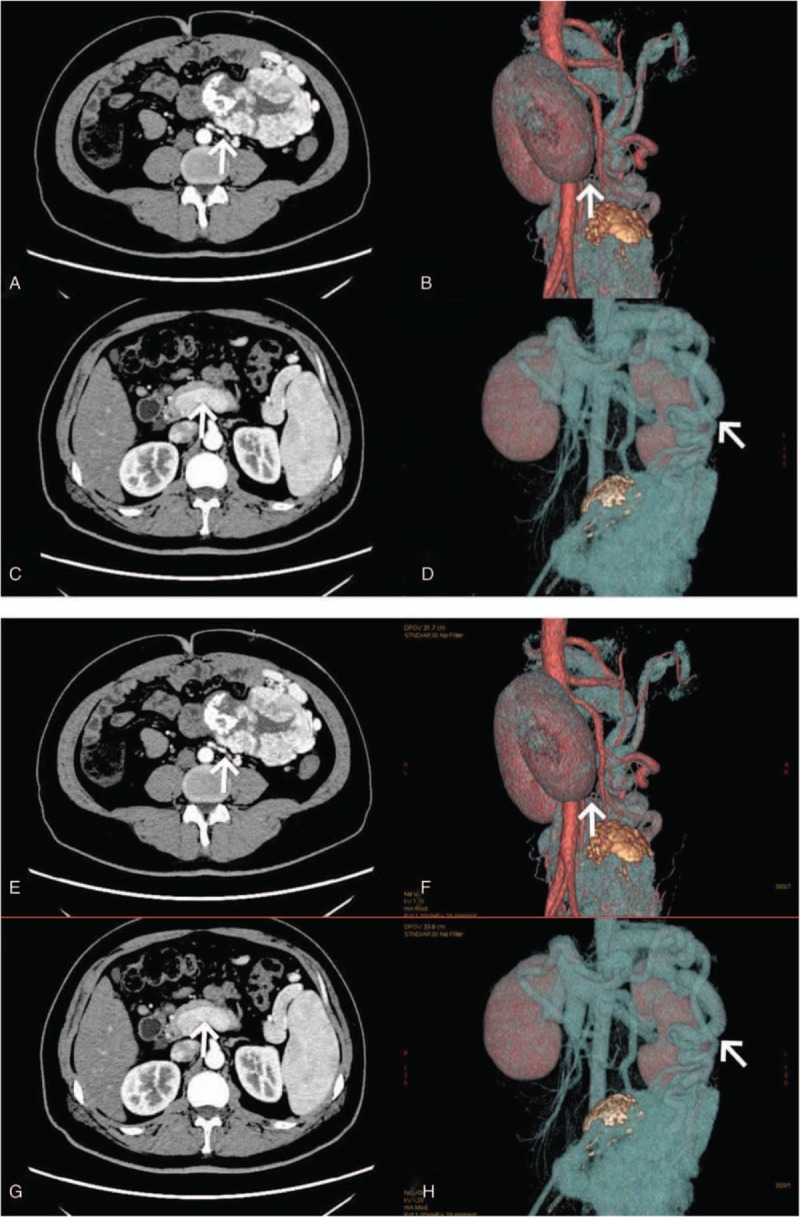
(A) The nourishing artery (arrow) of solitary fibrous tumors It is independently originated from the abdominal aorta, and nourishes the tumor. (abdominal CT scan) (B): The nourishing artery (arrow) of solitary fibrous tumors demonstrated with abdominal 3D revascularization. (C): The vein (arrow) of solitary fibrous tumors is located behind the kidneys and is divided into 2 branches which directly afflux to the portal vein. (abdominal CT scan)(D): The vein return (arrow) of solitary fibrous tumors showed by with abdominal 3D revascularization.
